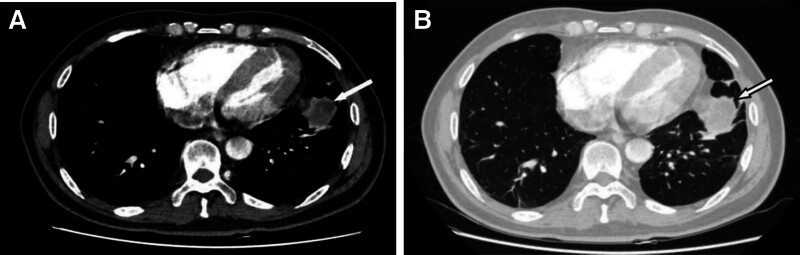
CT showed a mass in the left lower lobe. (A) The white arrow in the mediastinum window points to a mass in the left lower lobe, which has enlarged with marked heterogeneous enhancement and central necrosis. (B) The lung window reveals poorly defined margins and a concave adjacent pleural retraction. CT = computed tomography.
